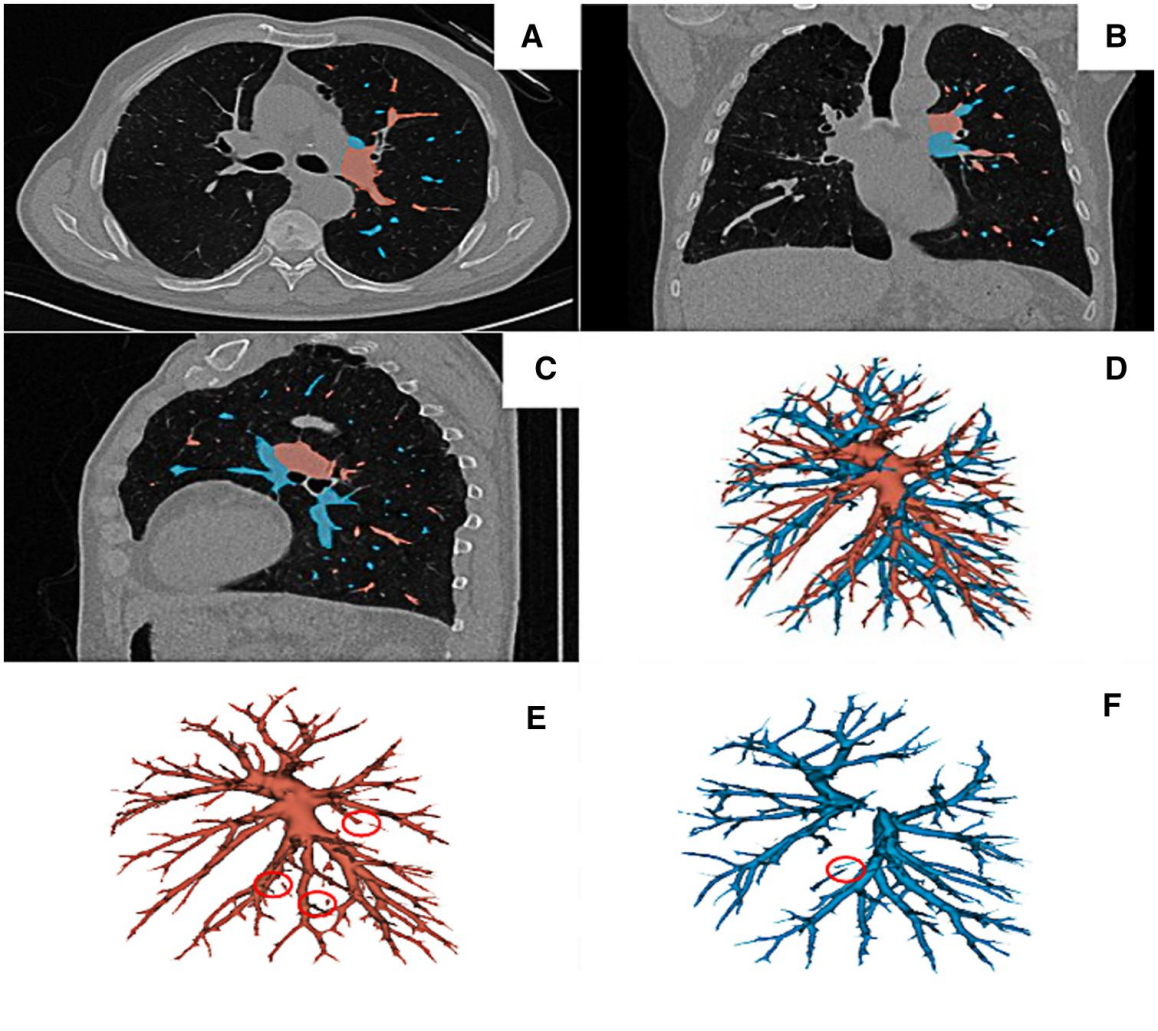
Overlap of automated segmentation of the left pulmonary artery (red) and the left pulmonary vein (blue) in axial view (A), coronal view (B) and sagittal view (C) on top of the CT scan. Full 3D visualization of the segmentation (D). Missing parts in the automatic segmentation for the left pulmonary artery (E) and left pulmonary vein (F) are highlighted with the red circles
